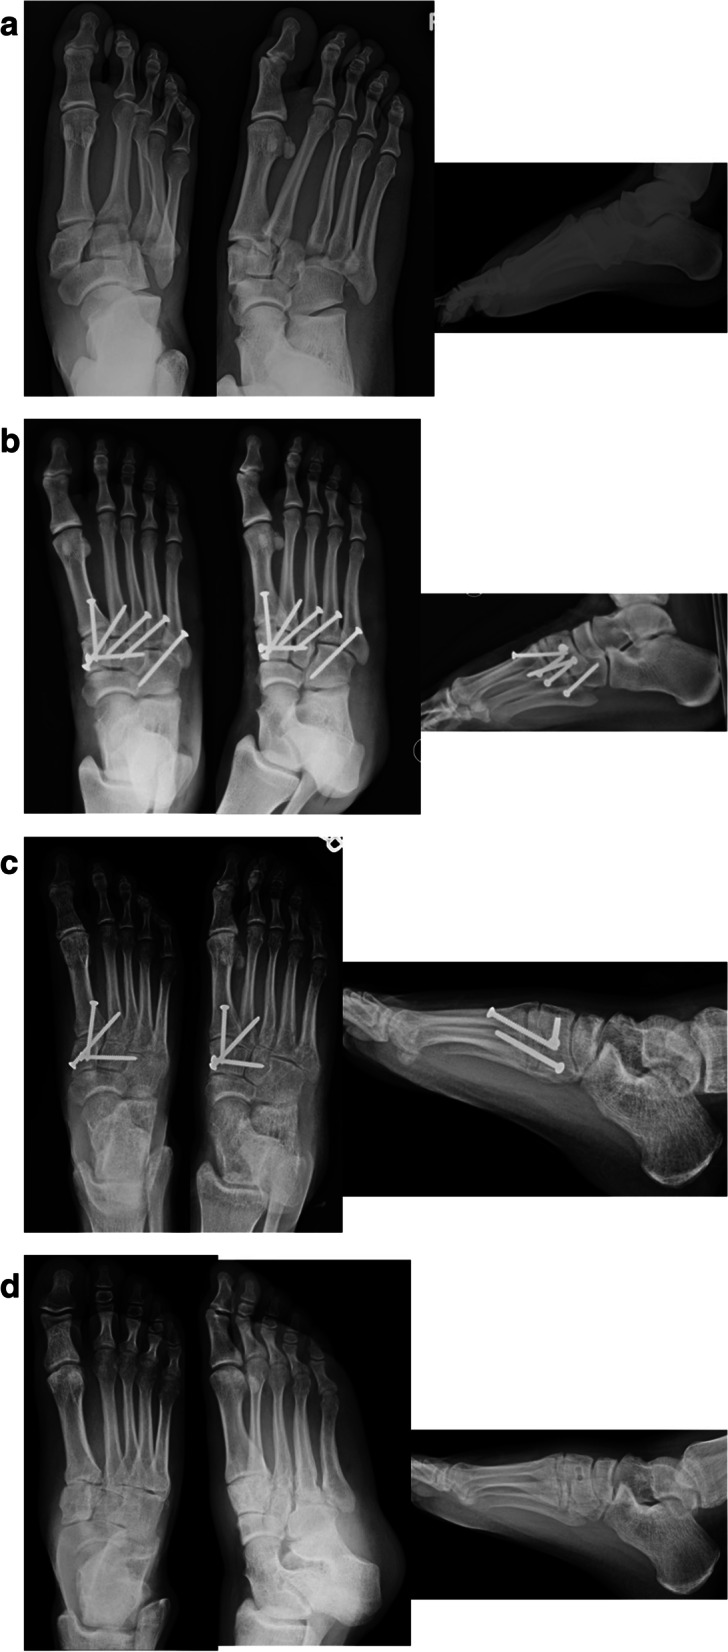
a Anteroposterior, oblique, and lateral injury radiographs of right foot demonstrating Myerson type C2 totally divergent TMT joint fracture-dislocation. b Anteroposterior, oblique, and lateral postoperative radiographs of right foot demonstrating restoration of anatomic alignment of midfoot and fixation across medial, middle, and lateral columns with cortical screws. c Anteroposterior, oblique, and lateral postoperative radiographs of right foot demonstrating removal of lateral column cortical screws and maintenance of anatomic alignment. d Anteroposterior, oblique, and lateral postoperative radiographs of right foot demonstrating removal of medial column hardware and maintenance of anatomic alignment
